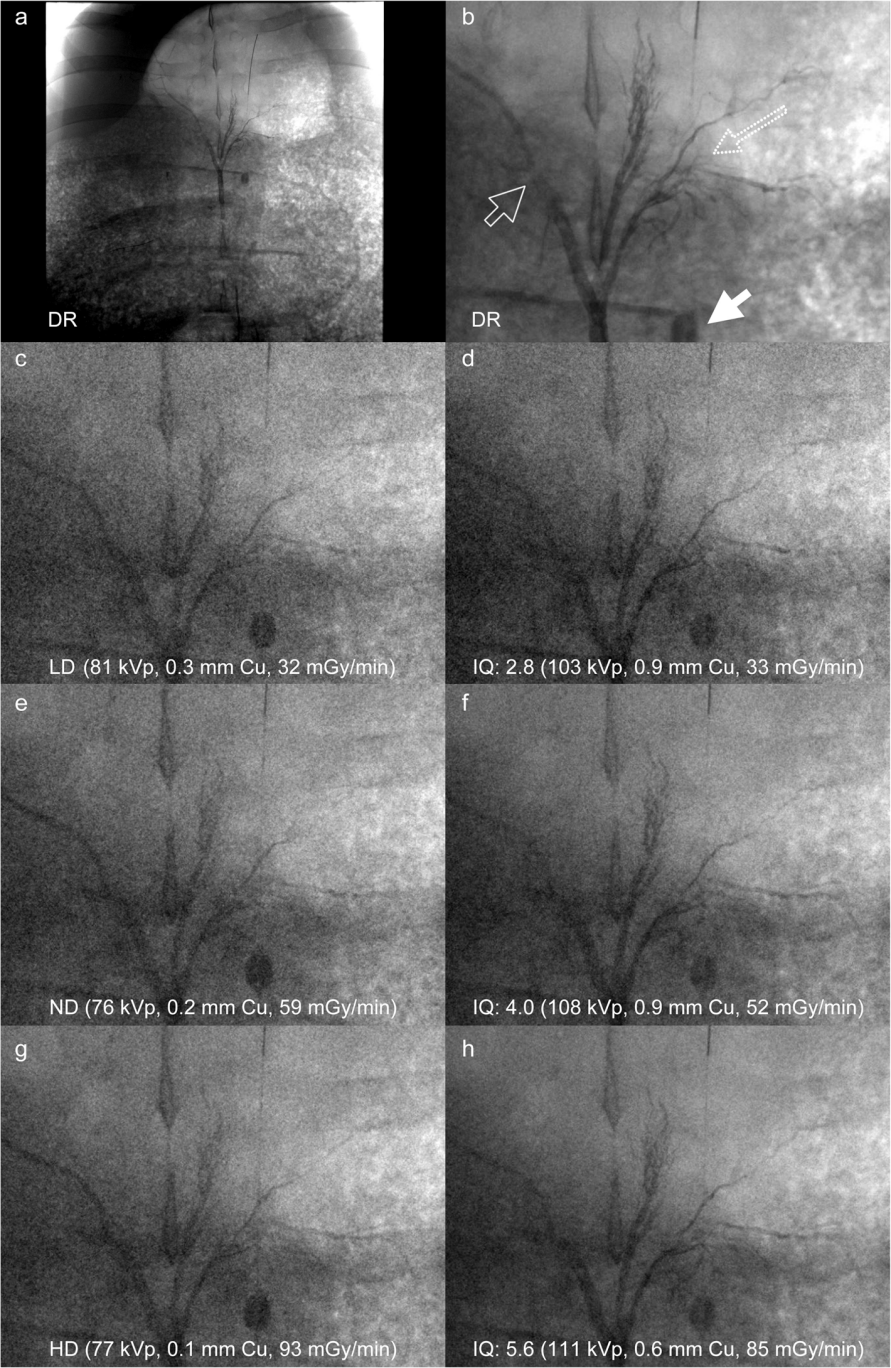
Example of fluoroscopic images of the liver at 32 cm water value acquired with the detector dose-driven exposure control using the three detector dose levels (LD low dose, ND normal dose, HD high dose) in comparison with fluoroscopic images acquired with the Ta-specific contrast-to-noise ratio-driven exposure control at a comparable incident air kerma rate (dose equivalent Ta image). The first row shows the overview image (a) and the magnified reference image (b), which were acquired with digital radiography (DR). The iodine mixed with cyanoacrylate-filled vessels and the iodine-filled balloon are indicated by the open and closed arrow. The dotted arrow indicates the tantalum filled gastric and liver arteries. *IQ* Image quality level
